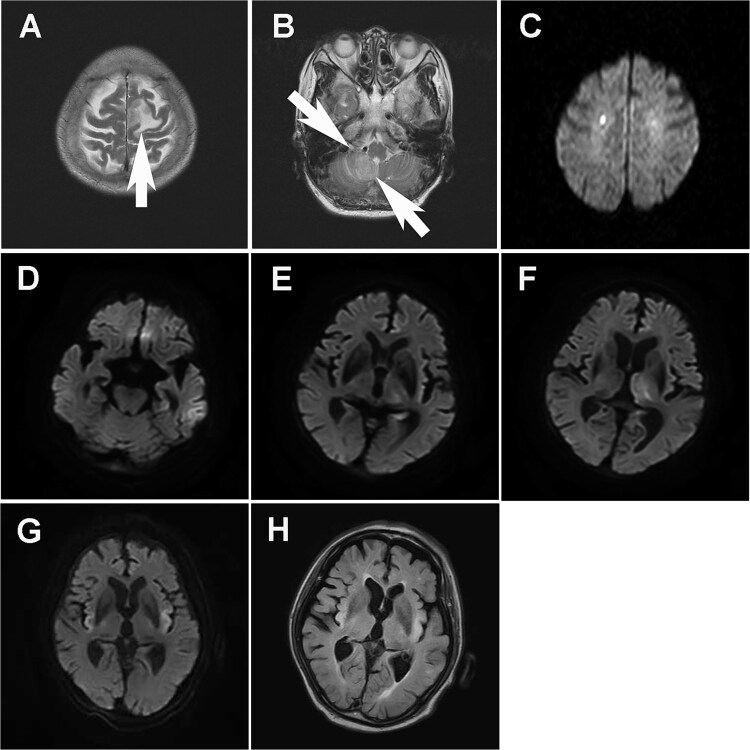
Brain MRI images. (A, B, C) case 1, (D, E, F) case 2, (G, H) case 3. (A, B) the edema surrounds the brain parenchyma in the right frontal lobe (arrow) and right cerebellar hemisphere (arrows). (C) DWI image shows acute lacunar infarction in the right frontal lobe. (D, E, F) DWI images show high signals in the (D) left parahippocampal gyrus and (E, F) left thalamus. (G) DWI image shows diffusion restriction, and (H) FLAIR image shows high intensity in both sides of the insular gyrus. The lesions are asymmetric.
